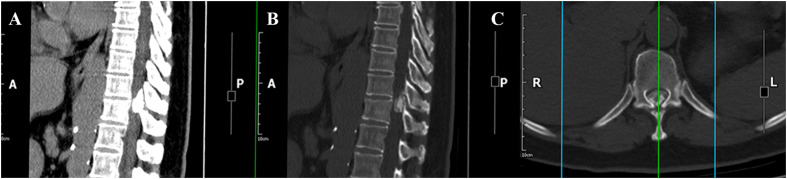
CT revealed complete ossification of the mass. (A) Sagittal CT image on bone window; (B) Sagittal CT image on soft-tissue window; (C) Axial CT image.
